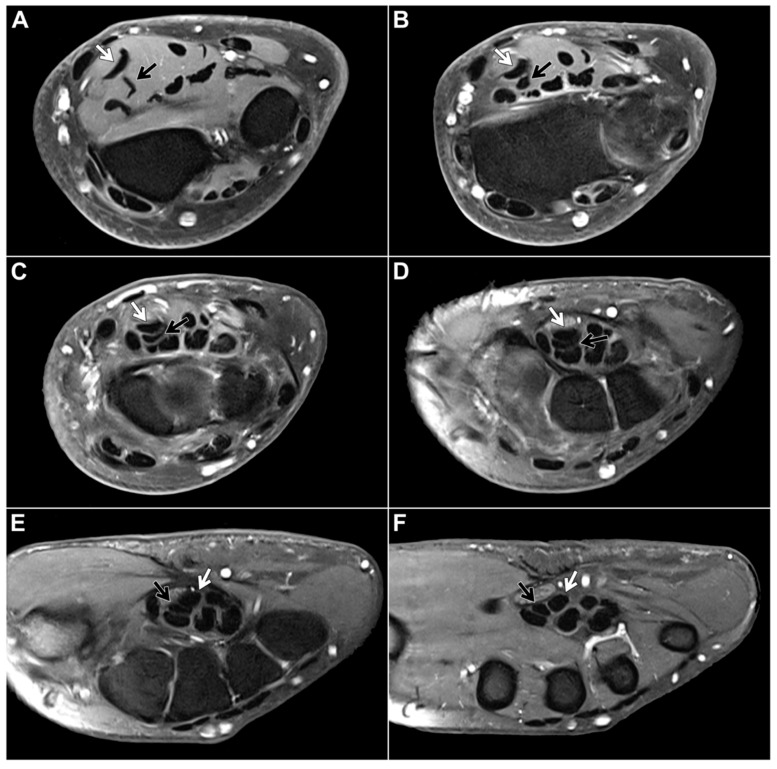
Fat-suppressed T1-weighted left forearm scan after intravenous administration of gadolinium-based contrast agent in a 48-year-old woman for presumed compression of the median nerve, which is located directly on the palmar side of the FDS tendons. Axial planes ((A,B): forearm level, (C–E): carpal tunnel level, (F): midcarpal level) depict the most common form of flexor digitorum superficialis anatomy: From the ulnar side of the forearm, the FDS/2 tendon (black arrow) crosses over the FDS/3 tendon (white arrow), thus forming the chiasma antebrachii.
